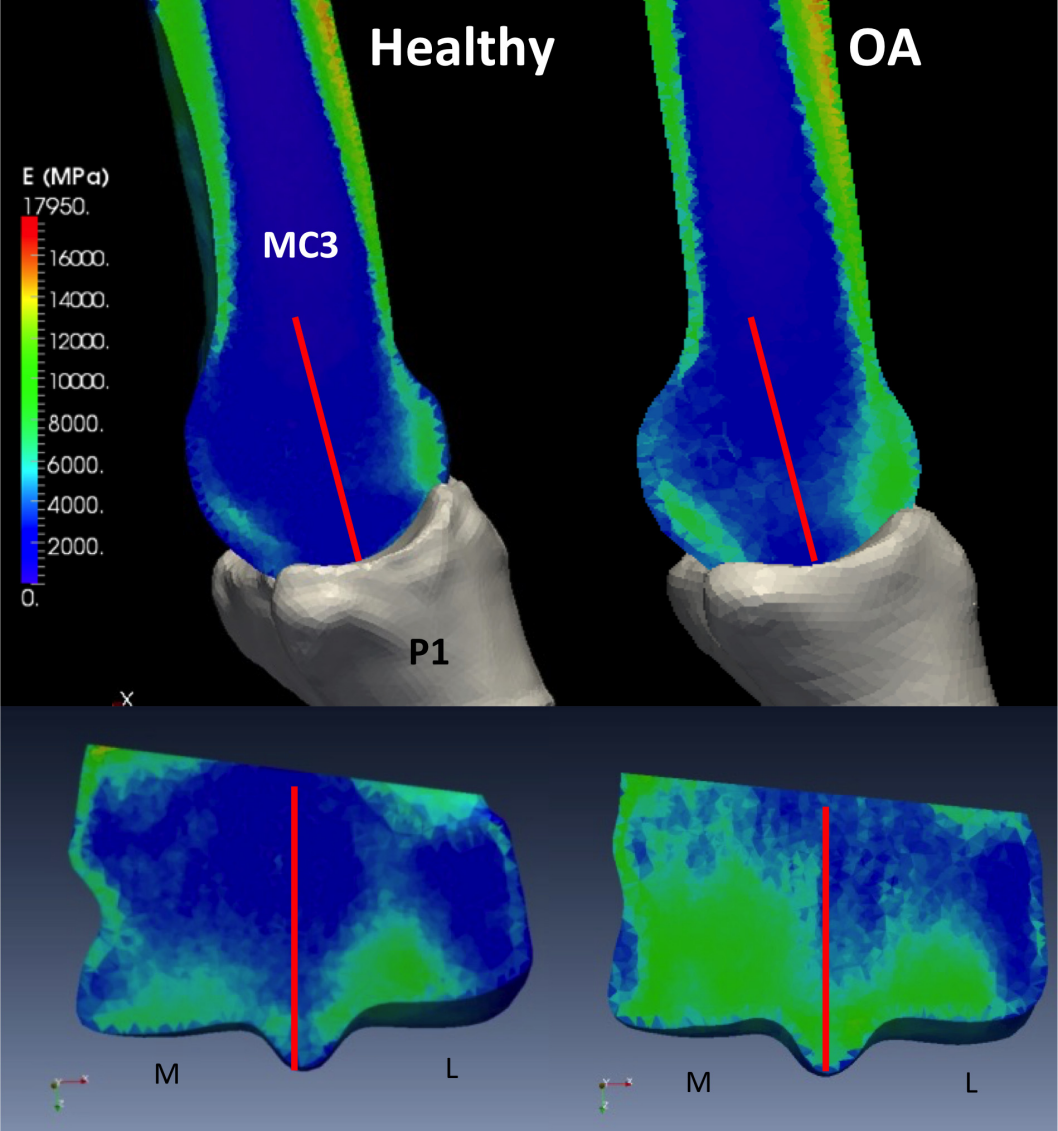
Mapped material stiffness on MC3 from μ-CT images. Upper images, parasagittal slices at locations indicated by a line on corresponding lower image. Lower images, frontal slices at locations indicated by lines on corresponding upper images. Bone stiffness represented by colour mapping on the images and corresponds to the colour scale which is measured in MPa.
